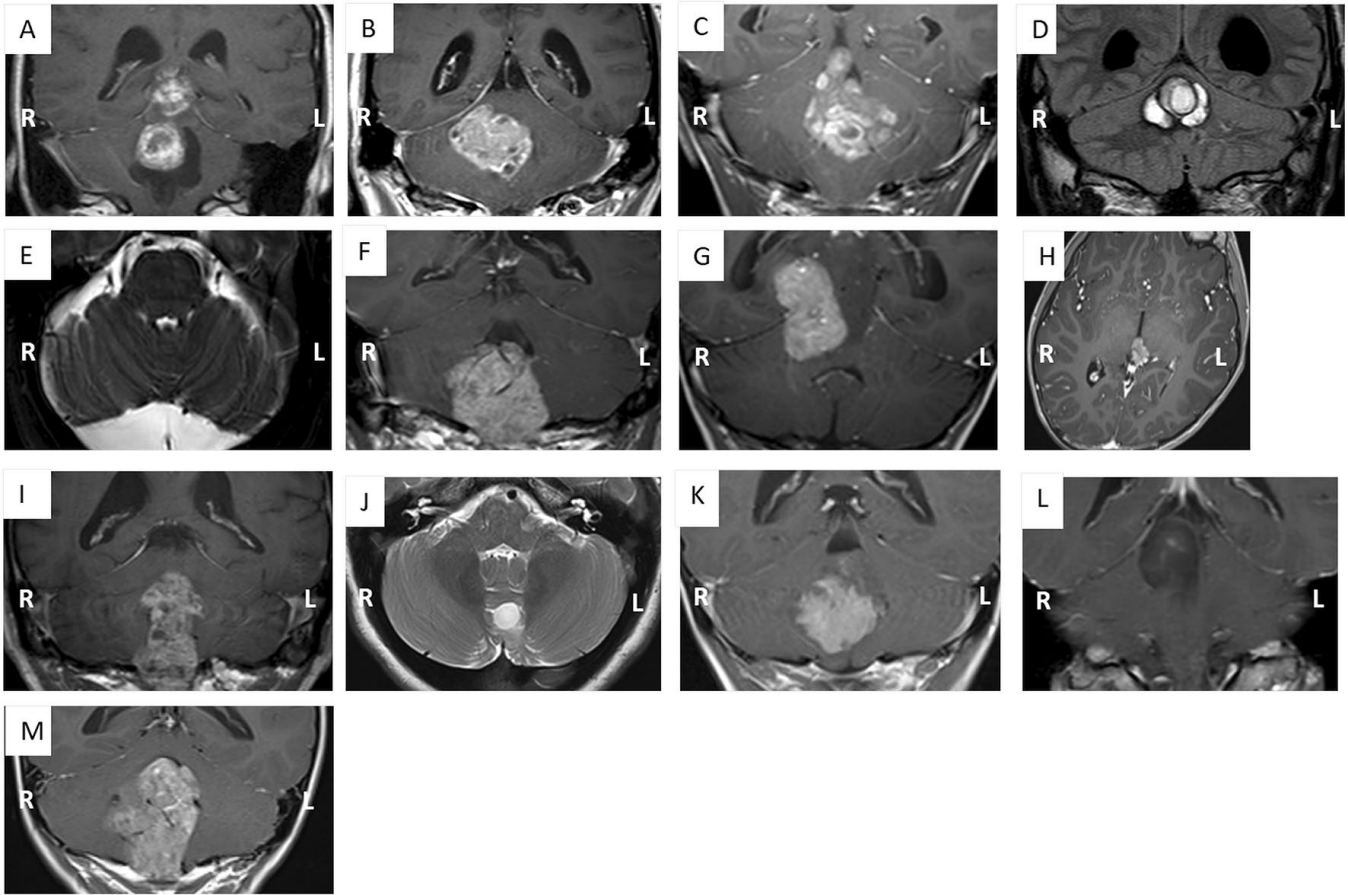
MRI scans demonstrating the pathologies of participants. (A) S1 ganglioglioma, (B) S2 metastasis, (C) S3 ependymoma, (D) S4 cavernoma, (E) S5 supracerebellar arachnoid cyst, (F) M1 ependymoma, (G) M2 pineoblastoma, (H) M3 pineoblastoma, (I) M4 ependymoma, (J) S6 pilocytic astrocytoma, (K) S7 ependymoma, (L) S8 diffuse astrocytoma, (M) M5 ependymoma.
